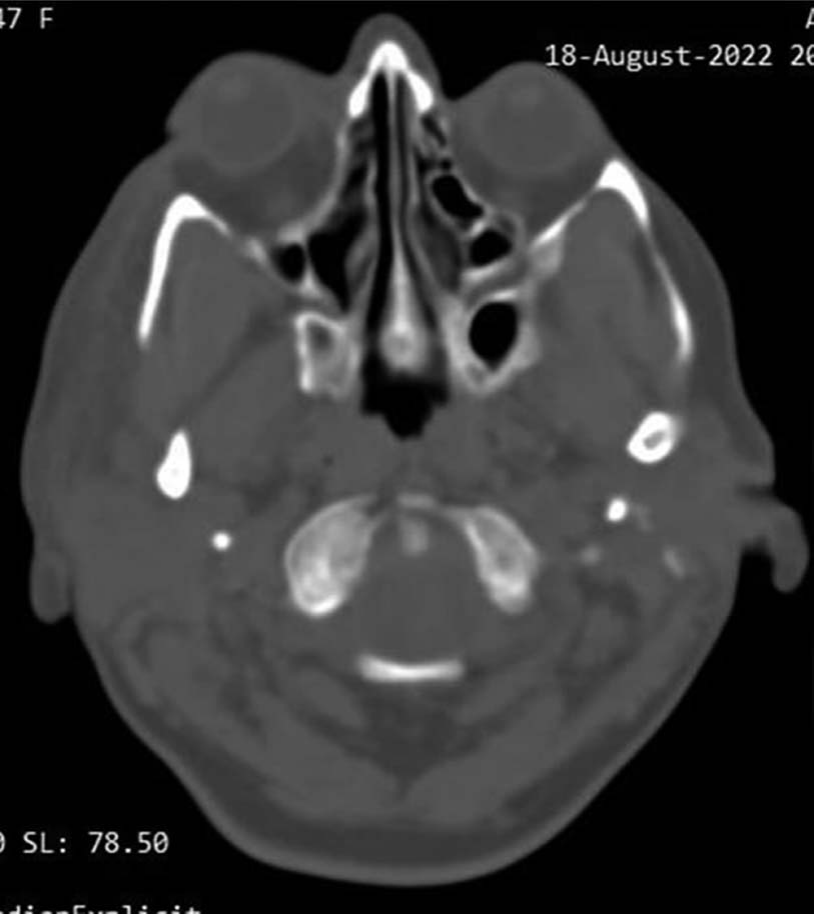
The computed tomography scan after 2 weeks revealed normal findings with no residues, and the proptosis significantly decreased.
